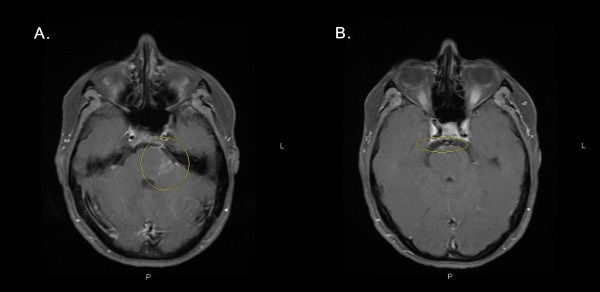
Brain MRI demonstrating basal meningitis during the patient's first admission. Axial T1-SE fat-suppression contrast-enhanced MRI scans showed abnormal signal intensity of the basal meninges, particularly involving the left cerebellar peduncle (A) and the right anterior mesencephalic-pontine junction (B).
